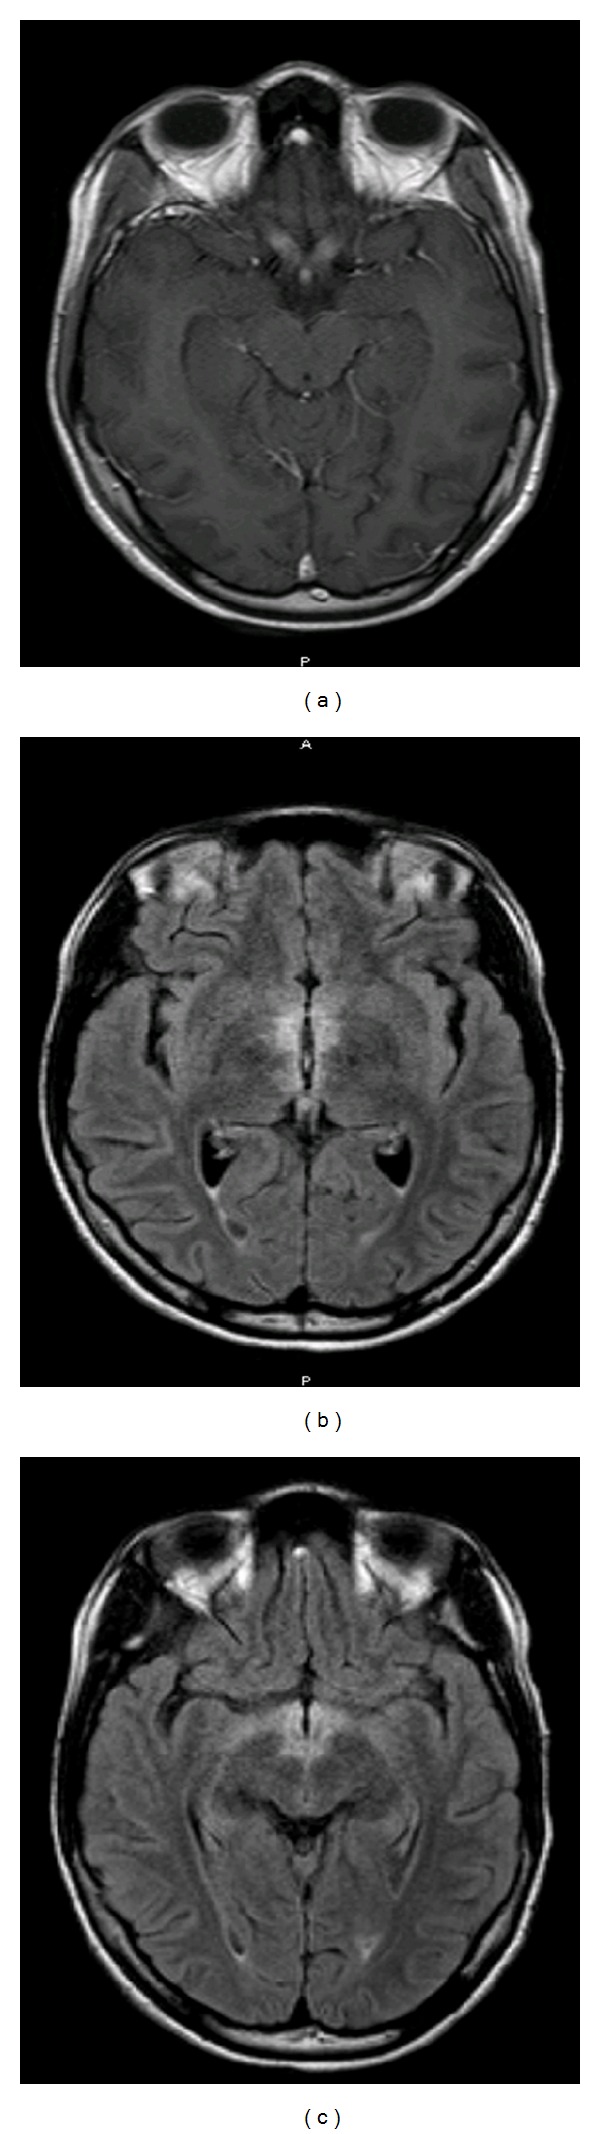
MRI of the brain: (a) axial T1W postcontrast: enhancement of bilateral optic nerves extending to the optic chiasm. (b) and (c): T2W FLAIR: increased signal in a symmetric fashion involving the hypothalami, mammillary bodies, periaqueductal gray matter, and posterior aspect of pons and medulla.
